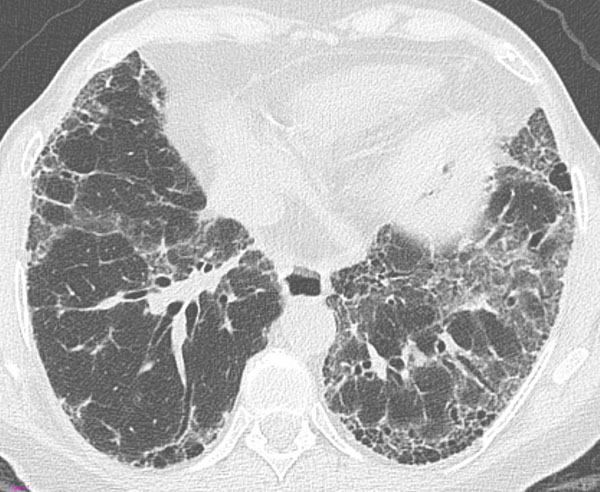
Definite UIP pattern. Transverse high-resolution computed tomography (HRCT) image shows subpleural basal honeycombing (more evident in the left lung) with traction bronchiectasis, reticular and ground-glass opacities.
